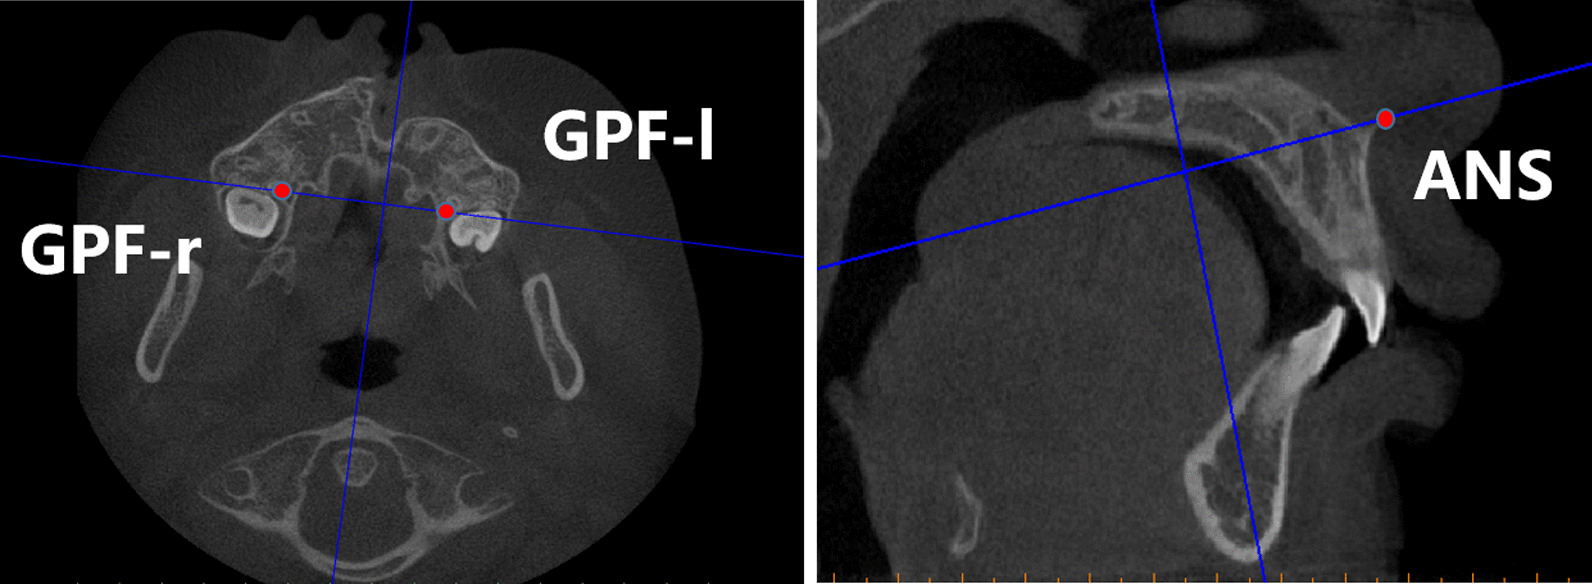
(Left) Greater palatine foramen point on the left side (GPF-L), greater palatine foramen point on the right side (GPF-R) and (Right) anterior nasal spinal point on the cone beam computed tomography axial and sagittal planes
